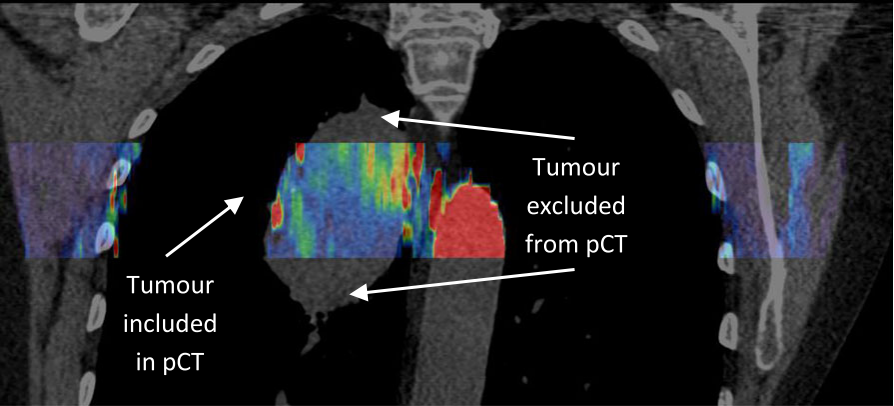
A coronal slice taken from one patient; the section in color indicates the volume over which the pCT was performed, while the underlying greyscale CT is from the pre-contrast CT
